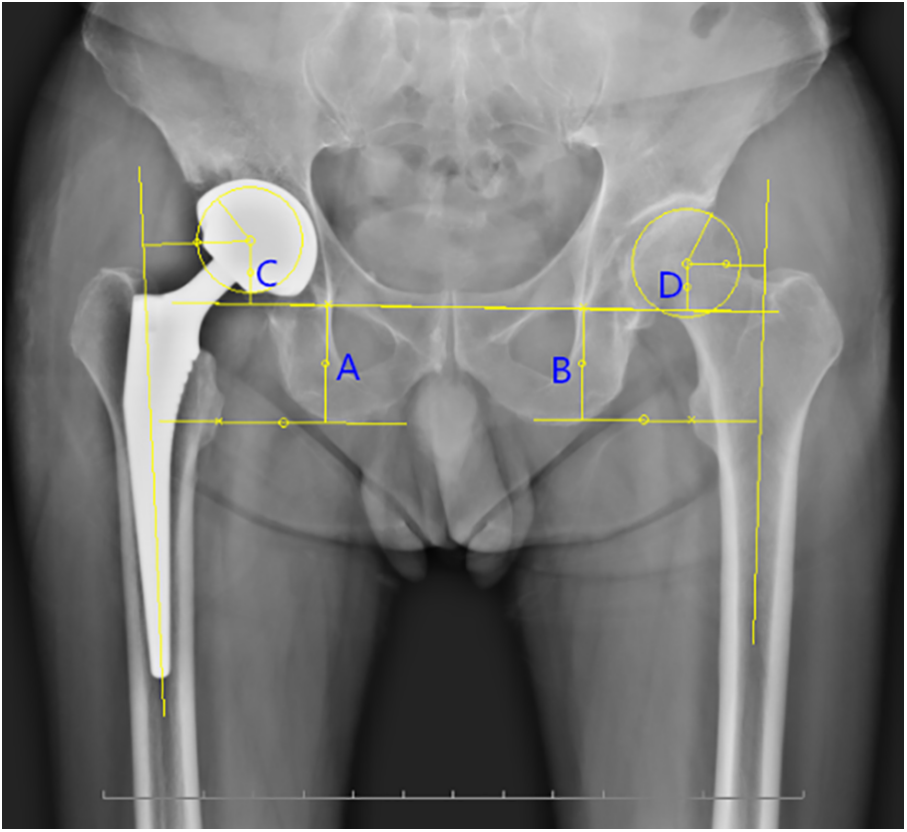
Measurement of the LLD and FO in the anteroposterior radiograph. LLD is defined as the absolute value of the sum of (A,C) minus the sum of (B,D). FO is the vertical distance from the rotation center of the femoral head to the long axis of the femur. FO, femoral offset; LLD, limb-length discrepancy.
